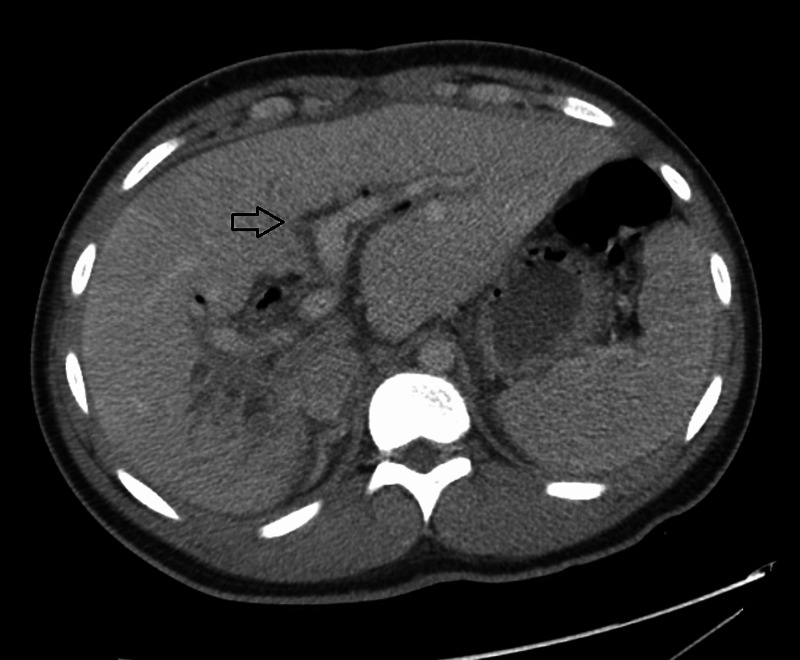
Abdominal CT scan showing multiple spheroidal foreign bodies within the dilated biliary tracts.
